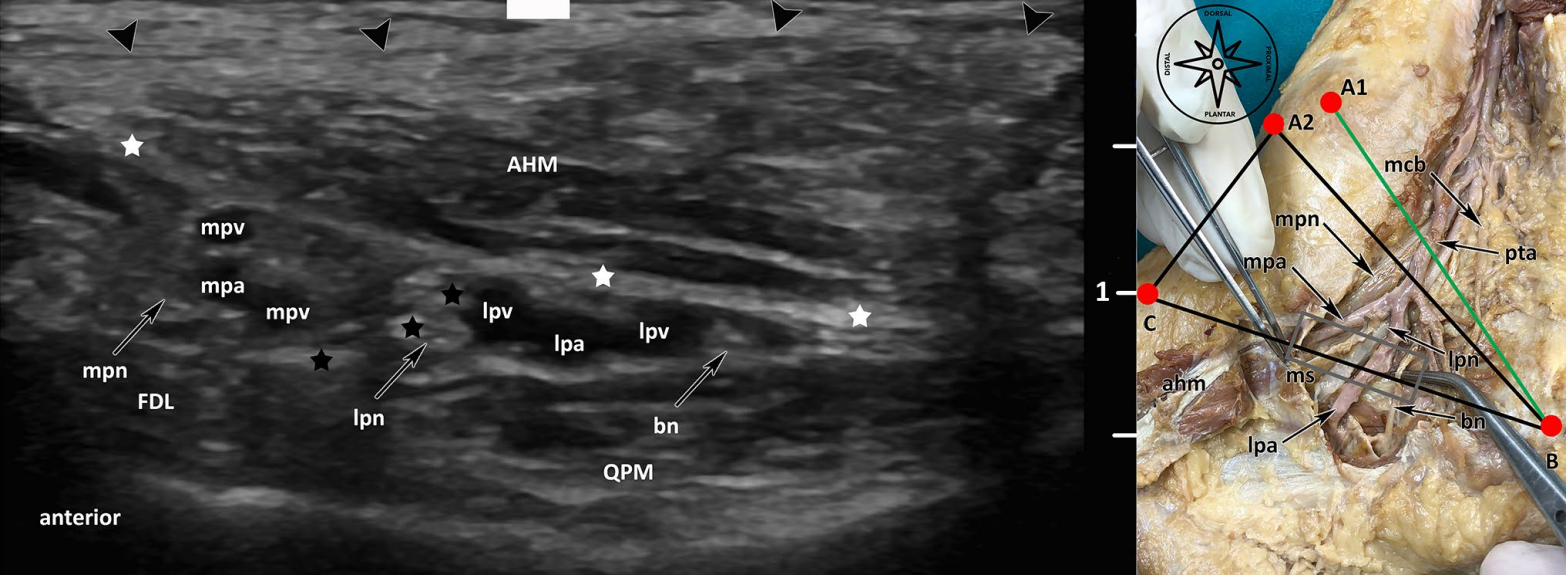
Ultrasound visualization of the terminal branches (lpn, mpn, bn) on the [BC] line. The figure on the right side shows an anatomical overview of the structures including the reference line “Dellon-McKinnon” (A_1_–B, malleolar-calcaneal) and the “Triangle of Heimkes” (A_2_–B–C). *Lpn* lateral plantar nerve, *mpn* medial plantar nerve, *bn* Baxter’s Nerve, *FHL* flexor hallucis longus muscle, *QPM* quadratus plantae muscle, *AHM* abductor hallucis muscle, *mpv* medial plantar vein, *lpv* lateral plantar vein, *mpa* medial plantar artery, *lpa* lateral plantar artery, *black arrow heads* superficial layer of the flexor retinaculum, *white stars* medial septum (deep fascia of the abductor hallucis muscle), *black stars* medial septum extension (“interfascicular septum”)
